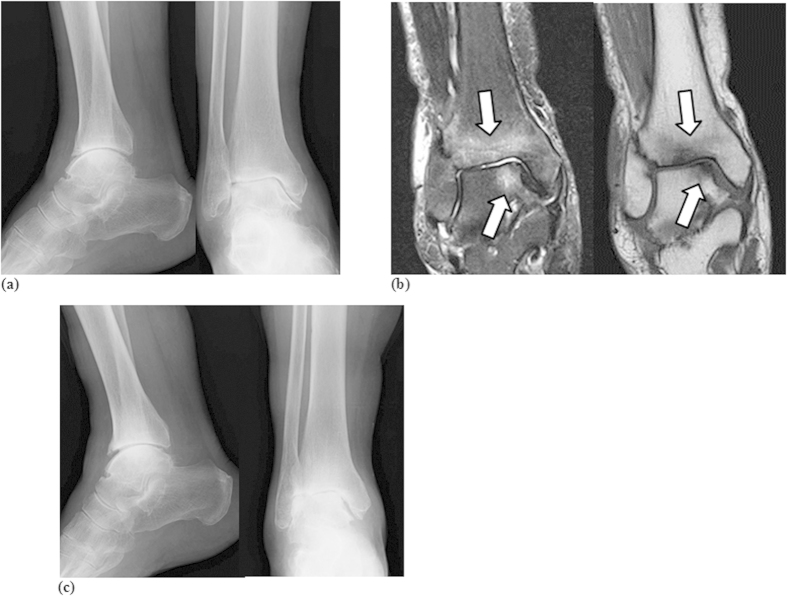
(a) Frontal (right panel) and lateral (left panel) plain radiographs showed mild joint space narrowing and osteosclerotic changes (KL grade IV). (b) Frontal T1W (right panel) and STIR (left panel) MR images. Bone signal changes depicted as low intensity by T1W and high intensity by STIR images along the talocrural joint line were evident (arrows). (c) Frontal (right panel) and lateral (left panel) views of plain radiographs showed increased joint space narrowing and osteosclerotic changes (KL grade IV).
